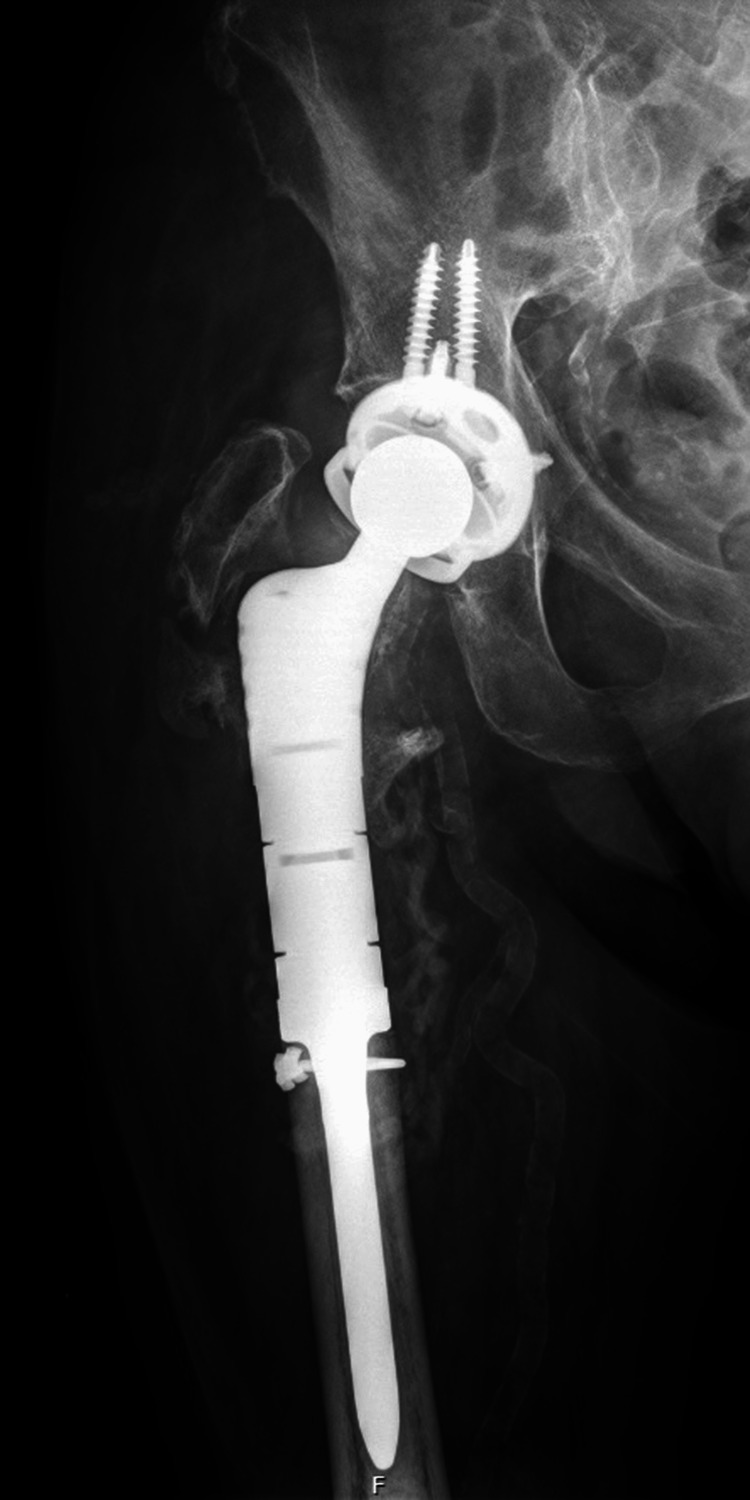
Six-week post-operative radiograph following right proximal femoral replacement Anteroposterior radiograph of the right hip and femur at six weeks post-operatively demonstrating a cemented DePuy Synthes modular proximal femoral replacement with an uncemented DePuy Synthes Pinnacle acetabular cup secured with screws. Implant alignment and positioning are satisfactory, providing a baseline for interval follow-up.
